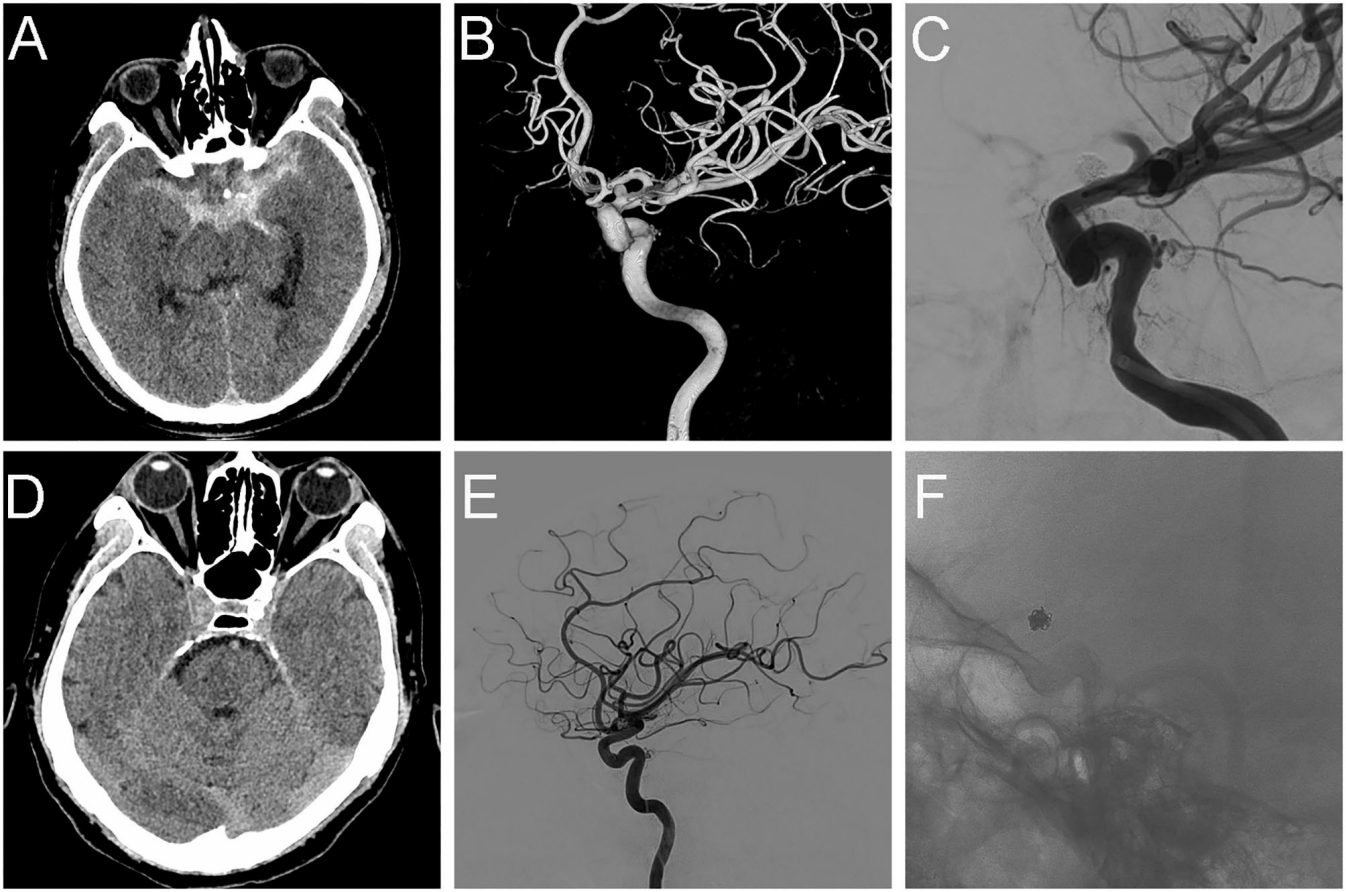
Typical case images. (A) CT scan showing SAH in suprasellar cistern before operation. (B) Preoperative DSA showing BBA at C7 segment of the left ICA. (C) Intraoperative DSA showing complete occlusion of the BBA using PED+ coils. (D) CT scan at 1-month follow-up. (E,F) DSA and Xper-CT at 6 months follow-up.
